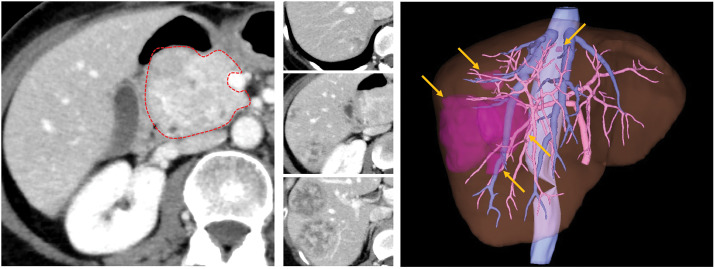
CT images of primary (red dotted line) and metastatic lesions (orange arrows) and 3D reconstructions by using SYNAPSE VINCENT (Fujifilm Healthcare Corporation, Tokyo, Japan). A primary lesion measuring 47 mm in diameter was observed in the head of the pancreas, showing contrast enhancement in the early phase. There were also 4 lesions in the posterior segment of the liver and 1 lesion in the root of the MHV. MHV, middle hepatic vein
